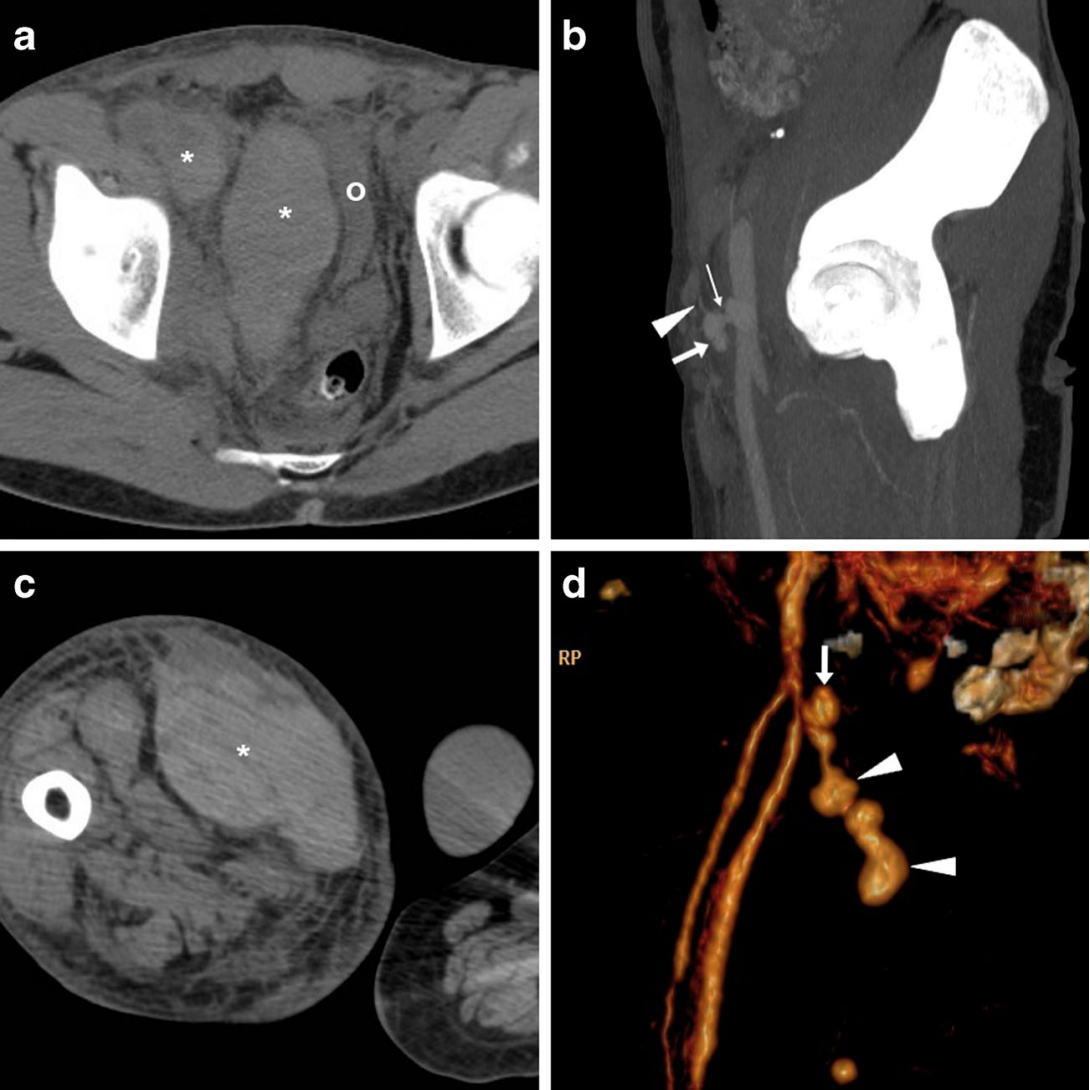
Two cases of right CFA PA complicated by bleeding. A-B) in a 29-year-old man on haemodialysis, suffering from pelvic pain four days after CA, precontrast (A) CT showed ample, high-attenuation retroperitoneal haematoma (*) compressing the urinary bladder (o). CT angiography MIP (B) showed PA (arrow) with thin feeding tract (thin arrow) and CM extravasation (arrowhead), which required immediate surgical treatment. C-D) In a 75-year-old man with myocardial ischaemia, 48 h after complex PCI CT detected a vast adductor muscle haematoma (*) caused by a small CFA PA (arrow volume-rendering image D) with CM extravasation (arrowheads), which required surgical repair
